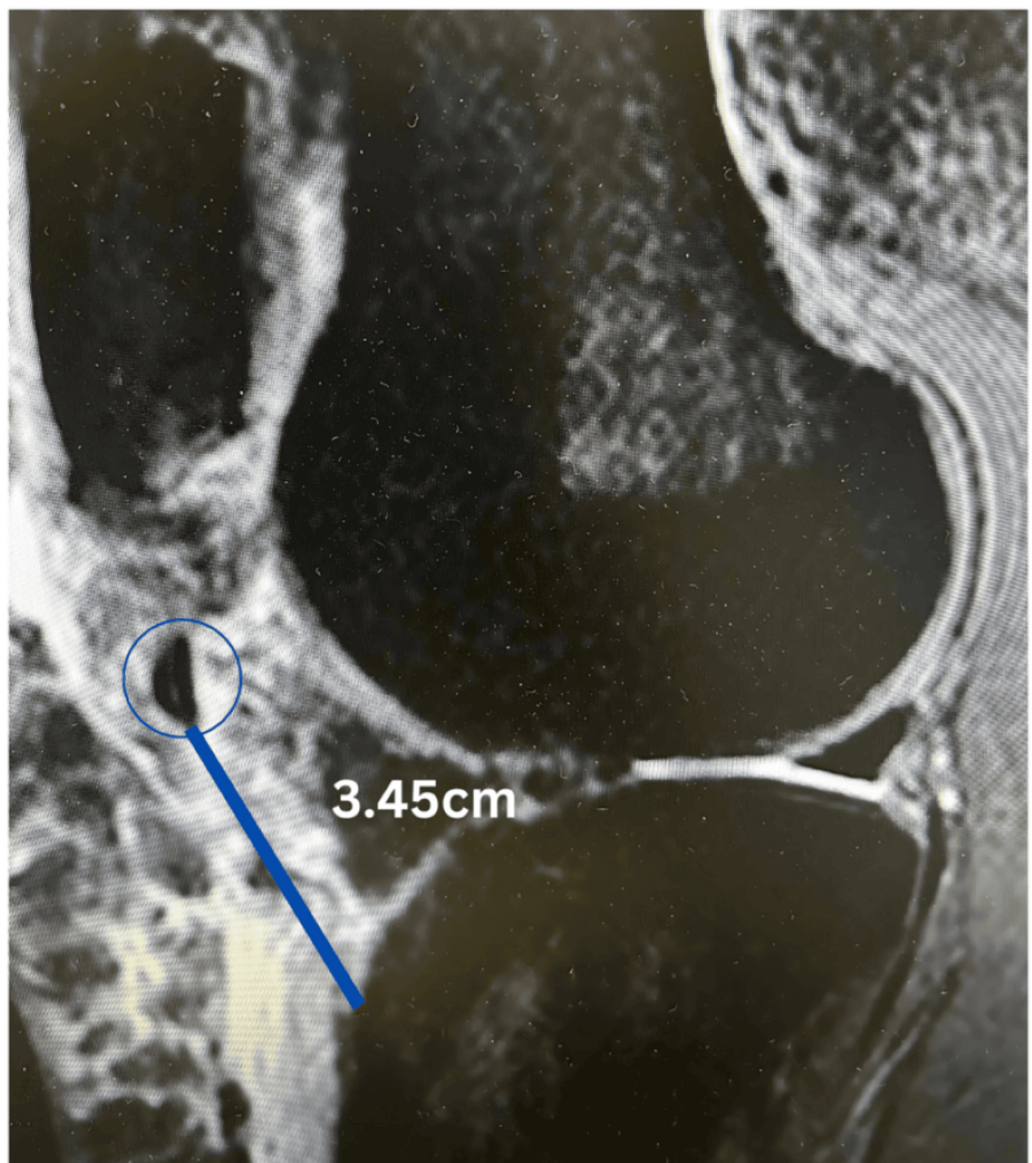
Initial MRI of the right knee MRI demonstrating a tibial tuberosity avulsion fracture (circled in blue) with retraction measured at approximately 3.45 cm.
